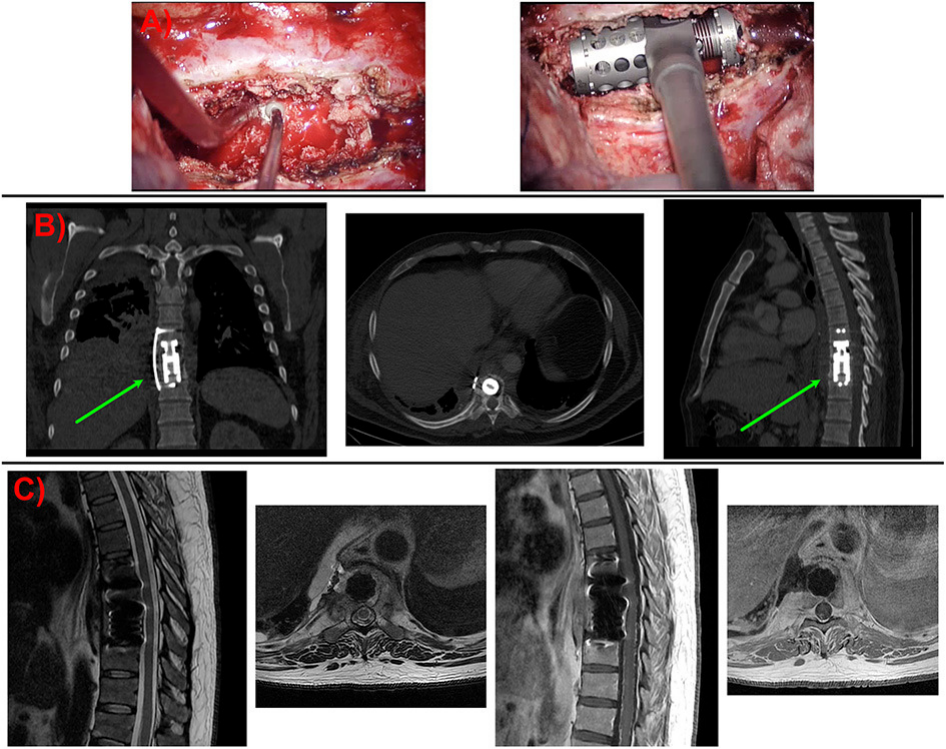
(A) Intraoperative images. Pathological bone in the affected vertebrae was drilled with a high-speed drill. Once the vertebrectomy was done, an expandable cage was fitted inside. (B) Coronal, axial, and sagittal CT views after surgery. No complications related to the approach or osteosynthesis materials were identified. (C) MRI images were taken after surgery. Note the evacuation of the epidural mass and the decompression done on the spinal cord in the axial views. Green arrow, osteosynthesis material (expandable titanium cage with a lateral fixation plate).
